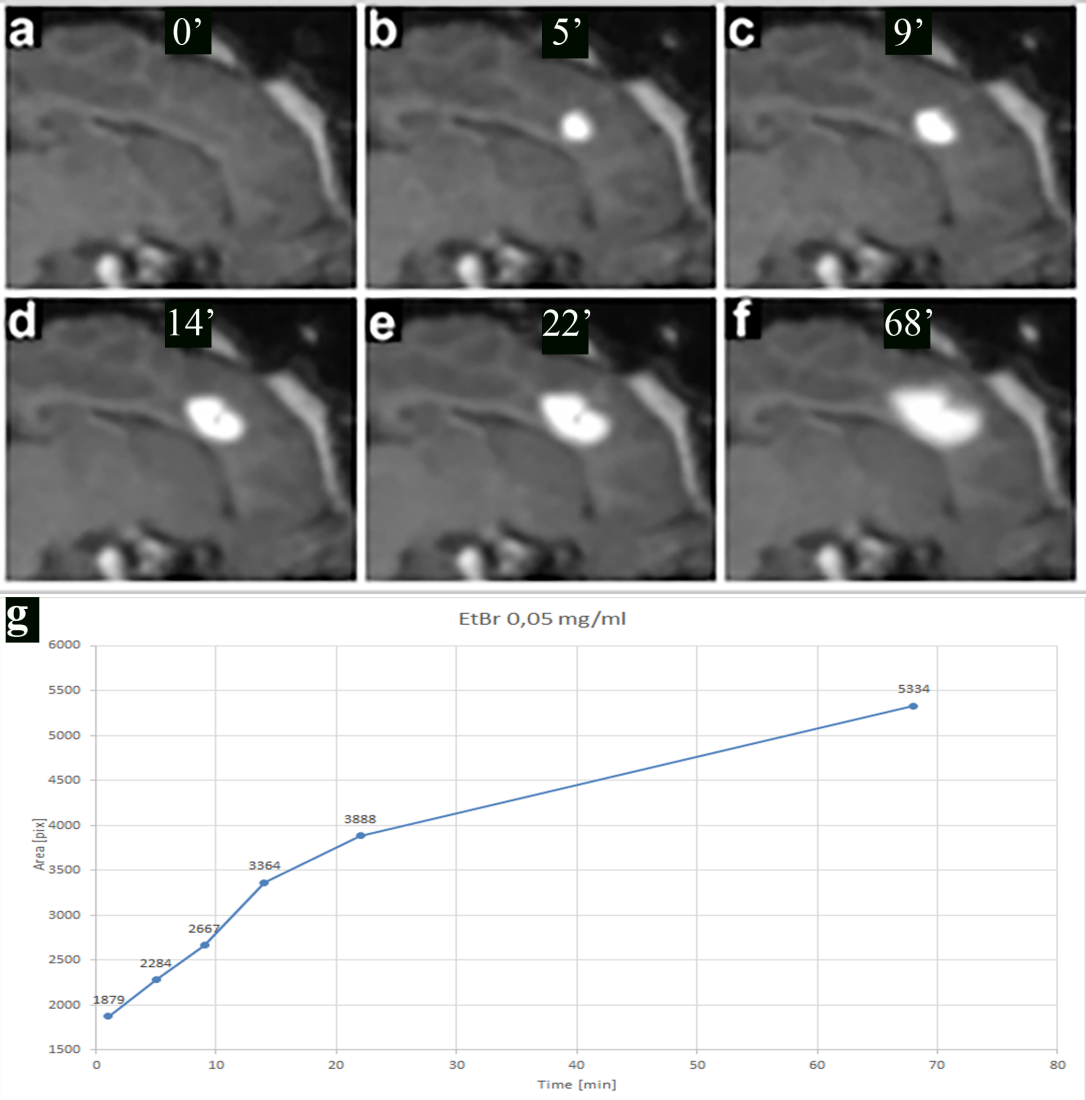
Convection-enhanced delivery of Gadolinium-supplemented gliotoxin. T1-weighted images were taken before (a), during (b-e) and directly after injection (f). Graph (g) is showing gliotoxin distribution over time during CED.
